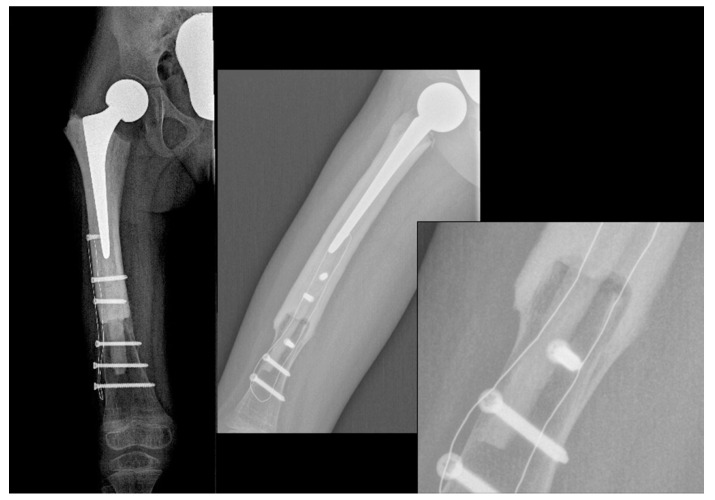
X-ray showing a stable fusion between the allograft and the femur.
